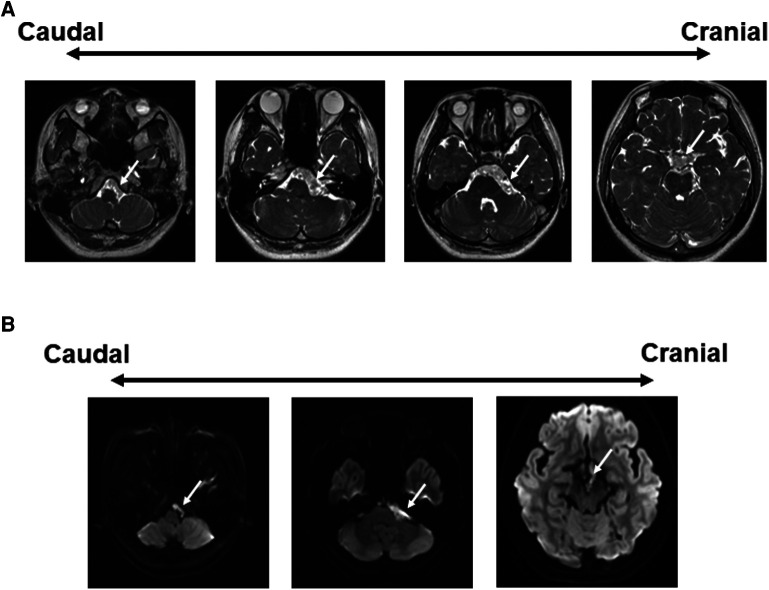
Preoperative radiographic findings. (A) FIESTA demonstrates an extra-axial lobulated mass at the left CPA, extending to the prepontine/interpeduncular cisterns as well as the ipsilateral FM, compressing the brainstem, and cranial nerves. There is also an extension into the ipsilateral IAC with encasement of the basilar artery. White arrow: tumor. (B) Axial DWI image shows bright signals in the CPA cistern. CPA, cerebellopontine angle; FM, foramen magnum; IAC, internal auditory canal.
